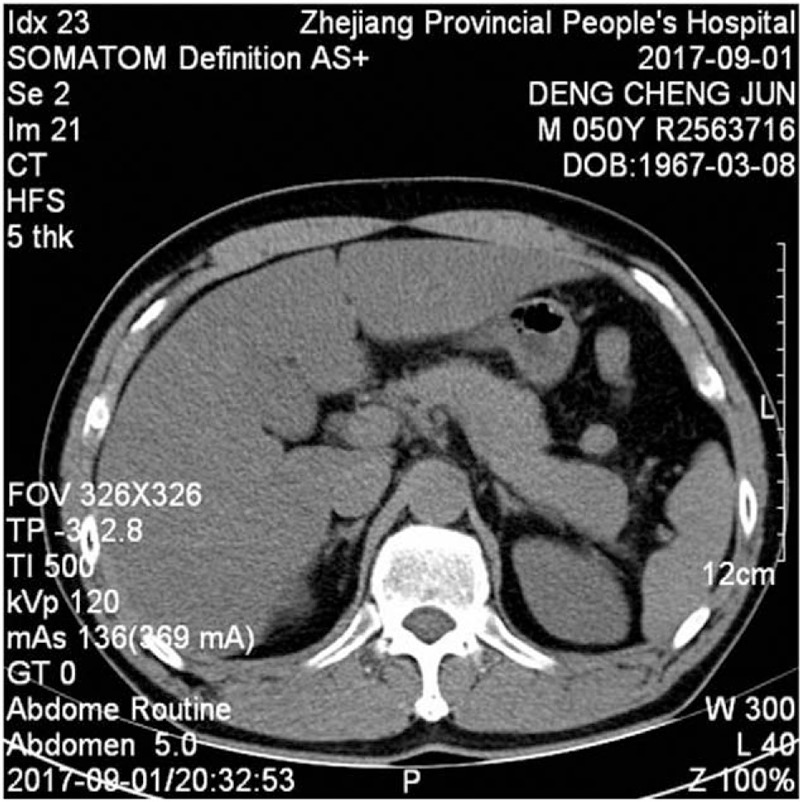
Abdominal computed tomography, with no fluid in the abdominal cavity, no swelling in the pancreas and no expansion of the stomach.
